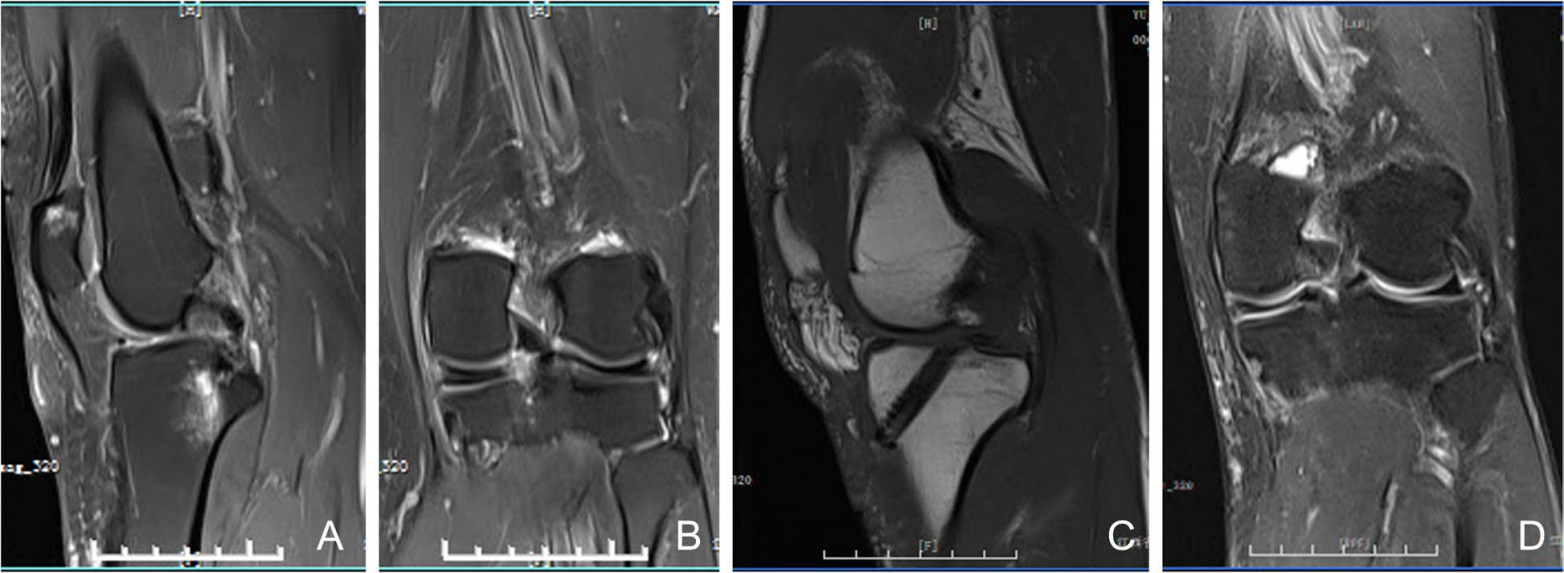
A 56-year-old male with a painful knee, A, B preoperative MRI study, Sagittal fat-suppressed proton density-weighted image (A) showing MMPRT radial tear with ghost signs, Coronal fat-suppressed proton density-weighted image (B) showing MMPRT radial tear with bone marrow edema like signal, C, D postoperative MRI study after MMPRT reconstructed with a gracilis autograft. MM: medial meniscus; MFC: medial femoral condyle; MTP: medial tibial plateau; MMPRT: medial meniscal posterior root tear
